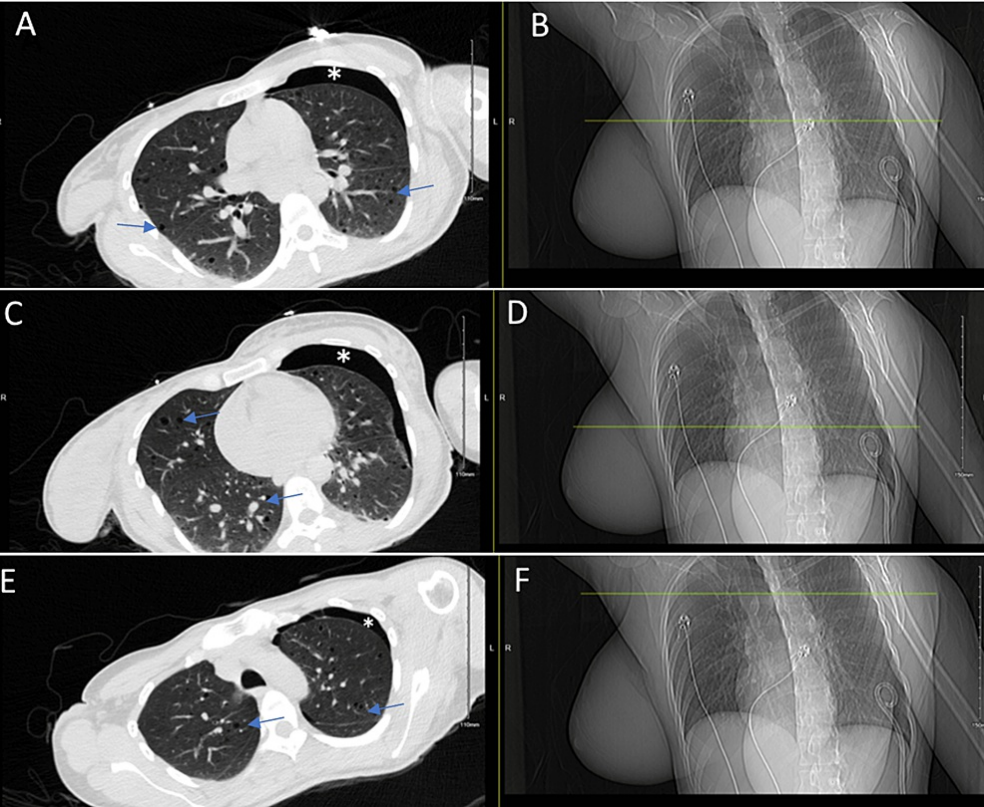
CT and corresponding Chest X-ray images CT imaging with corresponding Chest X-ray significant for left-sided pneumothorax, thin-walled cysts (arrows) diffusely throughout lungs with ground glass background suspicious for lymphangioleiomyomatosis. Area of pneumothorax highlighted by Asterisk (*). Figure 2A and Figure 2B demonstrate cysts and pneumothorax in the middle lung zones. Figure 2C and Figure 2D demonstrate cysts and pneumothorax in lower lung zones. Figure 2E and Figure 2F highlight cysts and pneumothorax in upper lung zones.
